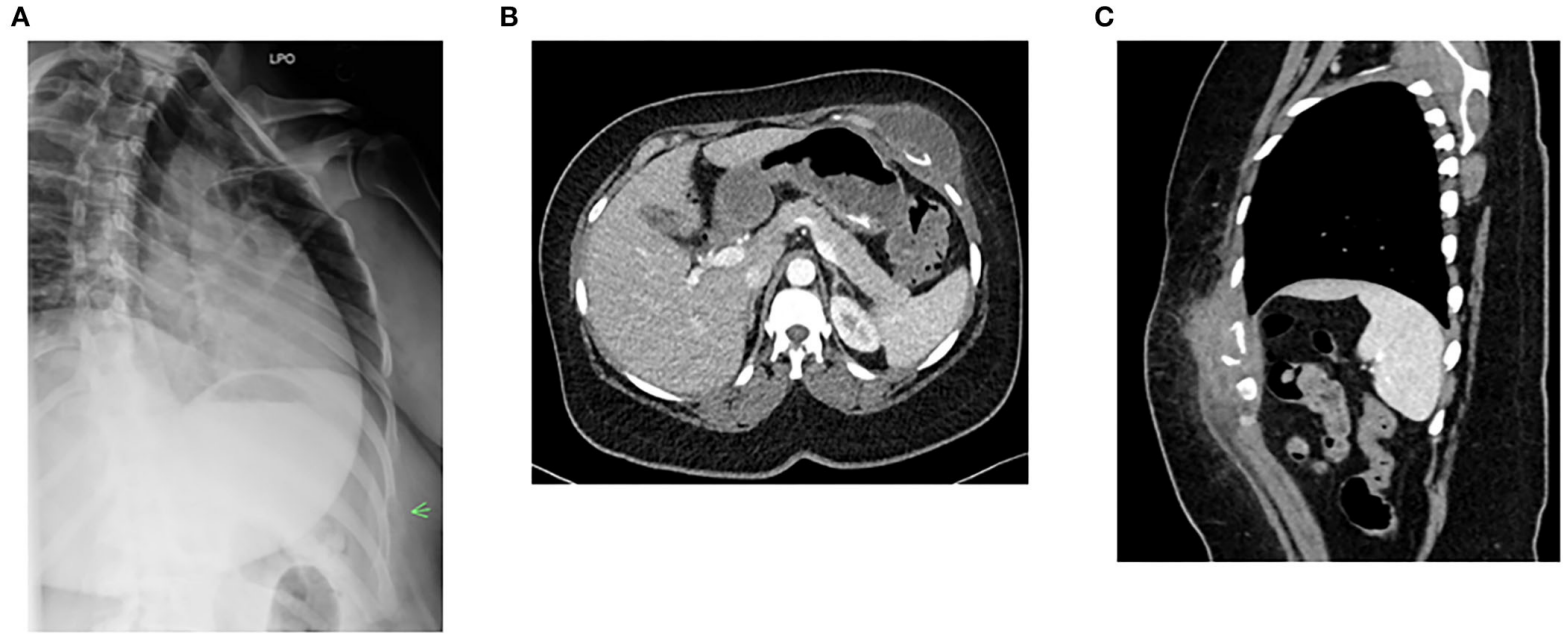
Imaging findings of chest wall mass and rib osteomyelitis. (A) Chest X-ray shows destruction of a rib. (B) Left chest wall mass (axial image). (C) Left chest wall mass (coronal view). Green arrow indicates the level of 7th rib fracture and mass.
